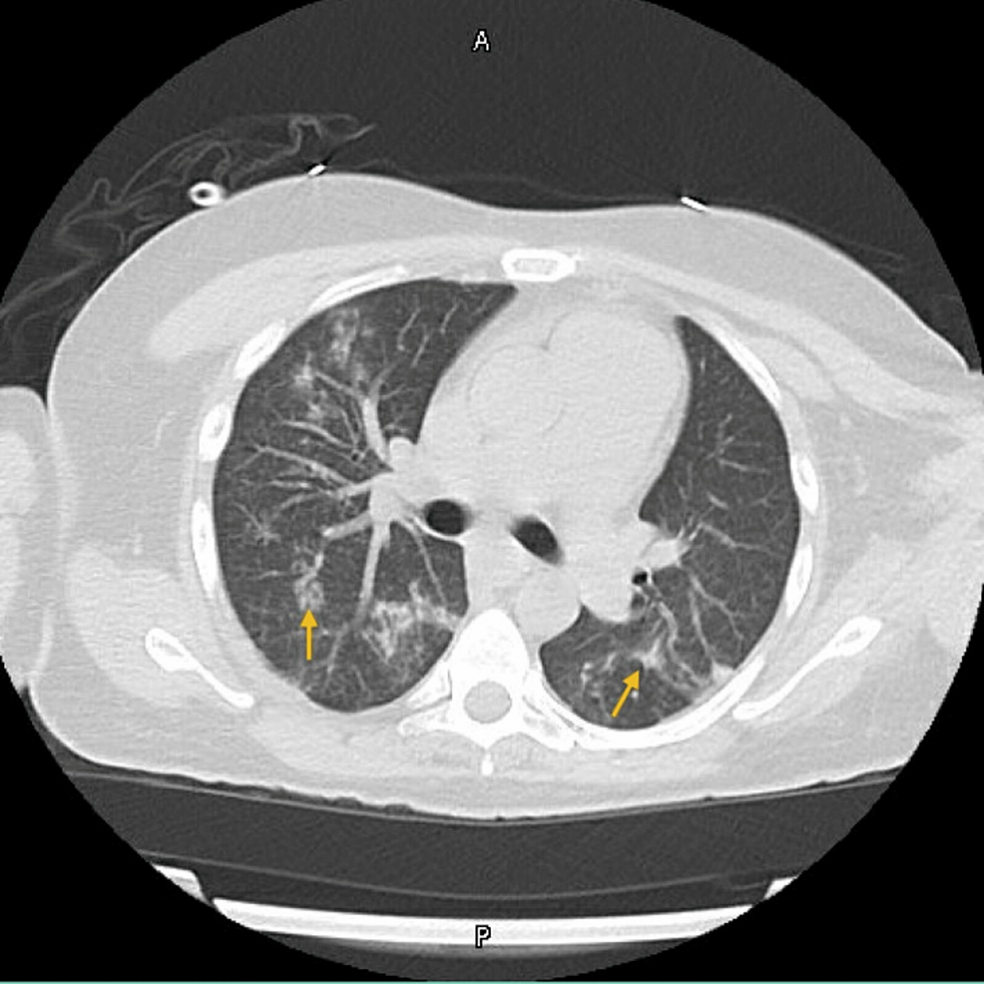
CT chest showing nodular infiltrates concerning for septic emboli (arrows) CT: computed tomography
